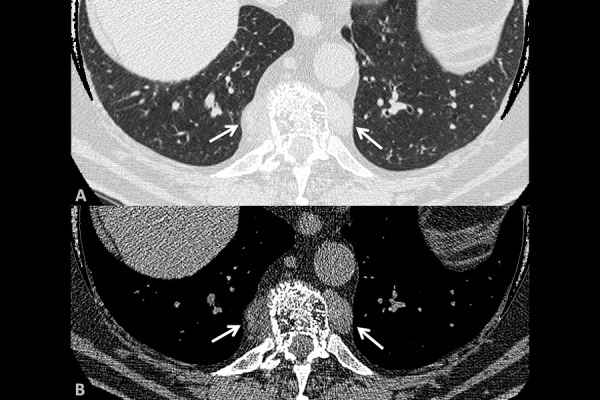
Axial view of HRCT shows two masses (white arrows) occupying left and right paravertebral mediastinal regions.
